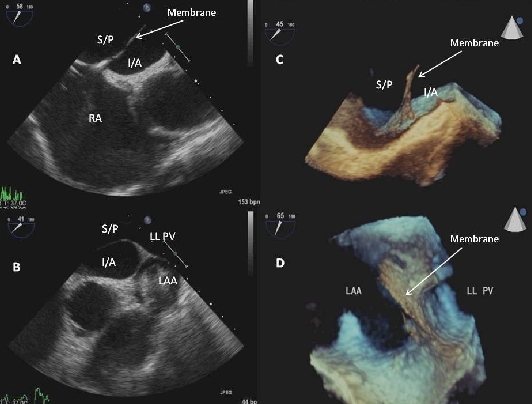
Standard transesophageal pictures showing septal insertion of membrane posterior to fossa (A) and lateral insertion at venous-appendage ridge (B). Panels C and D show insertion points on 3-Dimensional TEE imaging. Panel C shows fenestration of membrane and D demonstrates anatomical separation of appendage (LAA) and left lower pulmonary vein (LLPV) by membrane extending into left atrium. Abbreviations: S/P = Supero-Posterior left atrial chamber; I/A = Infero-Anterior left atrial chamber; LAA = Left Atrial Appendage; LLPV = Left Lower Pulmonary Vein.
